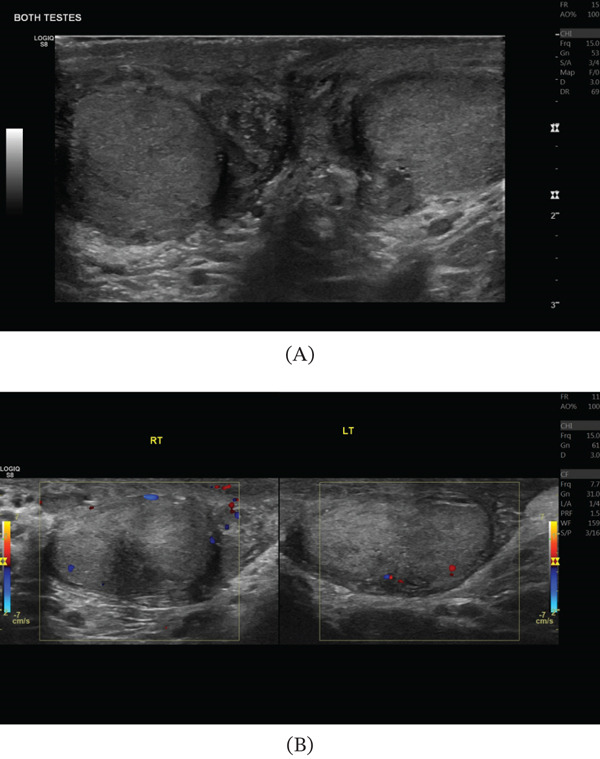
Six‐week postoperative ultrasound (US) of both testes; (A) conventional US of both testes and (B) Doppler US of both testes demonstrating reduced blood flow especially on the right and relative reduction in testicular volume to 3 cc bilaterally.
